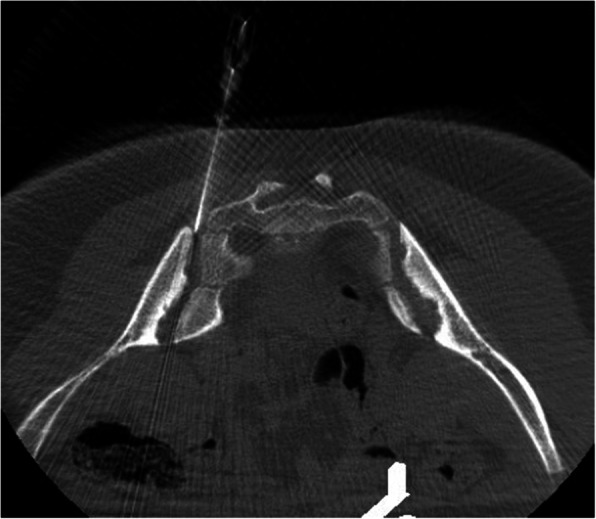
Cone beam CT guided SI joint injection w/o contract
